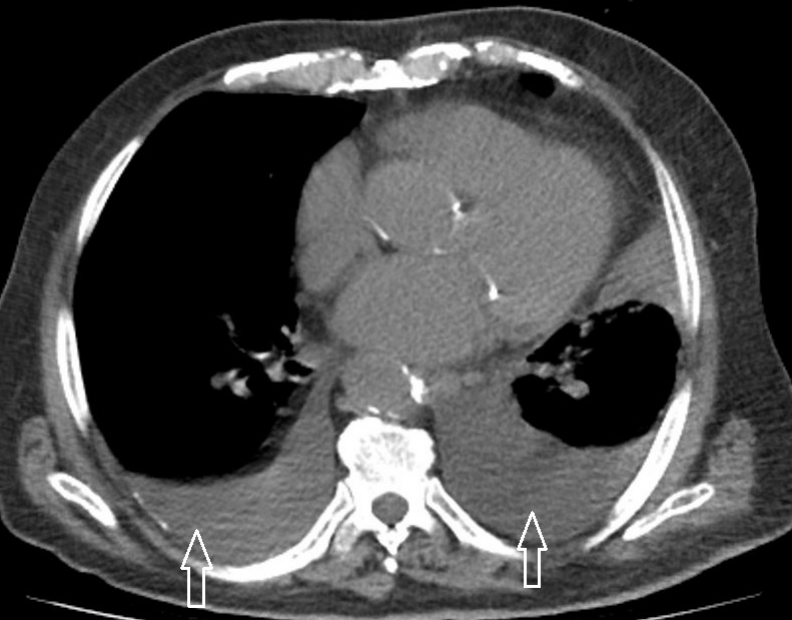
Significant pleural effusion was detected in the Chest CT of this patient (70 years age, male, with diabetes mellitus), and it was shown that the presence of pleural effusion was an important determinant of mortality in our patients over 65 years of age. White arrows: pleural effusion.
